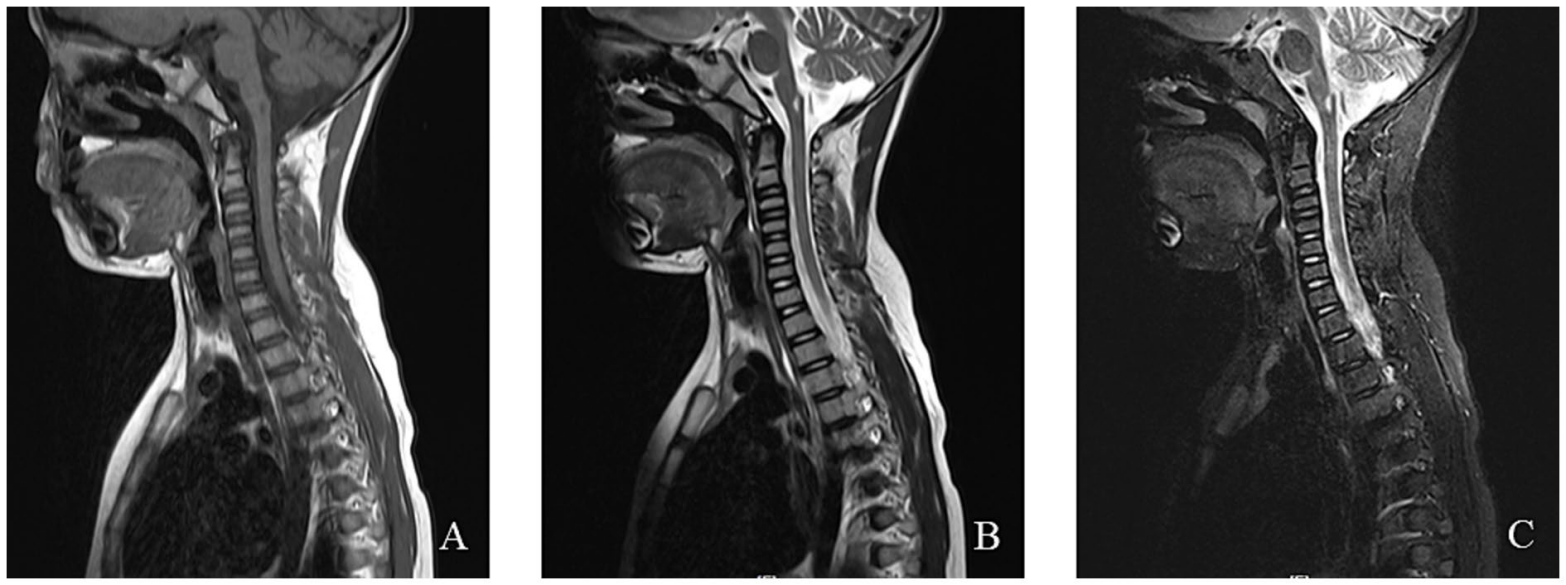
An 8-year-old female was diagnosed with MERRF syndrome due to limb tremors for more than 10 months. (A–C) Cervical MRI showed a patchy abnormal signal in the medulla oblongata. T1WI showed a low signal, T2WI showed a high signal, and the fat-suppression image showed a slightly high signal.
